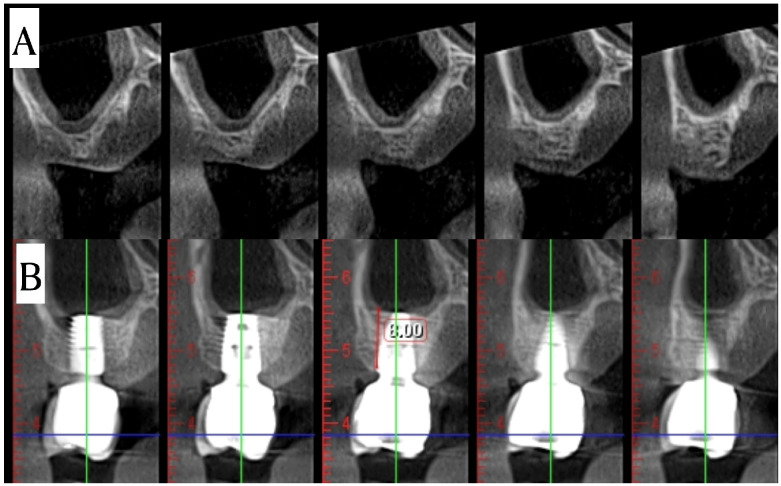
CBCT image of Case #5 at 10 months preoperatively (A) and postoperatively (B).
